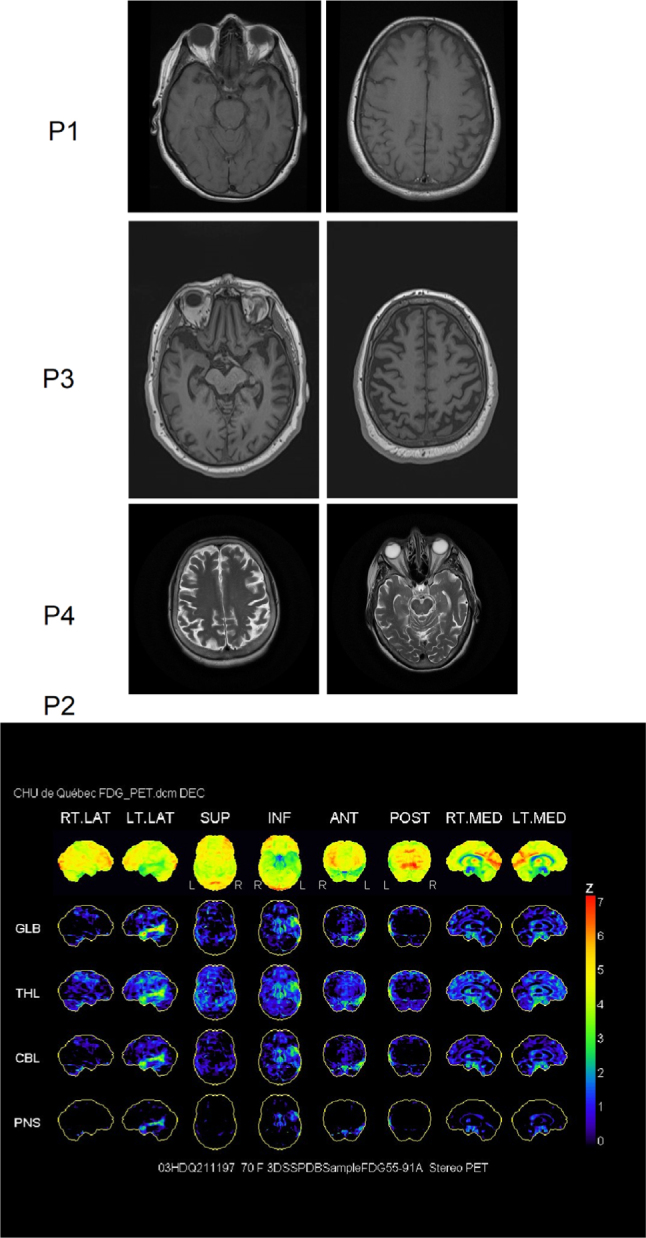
Transverse weighted MR images for P1, P3 and P4 and FDG-PET scans of P2. P1. Axial 3DT1 MRI showing left anterior temporal and left hippocampal (left image) and left parietal atrophy (right image). P3. Axial 3DT1 MRI showing left temporal (left image) and left posterior parietal atrophy (right image). P4. Axial T2 MRI showing left parietal (left image) and left temporal atrophy (right image). P2. 18F-FDG-PET showing left temporoparietal hypometabolism.
